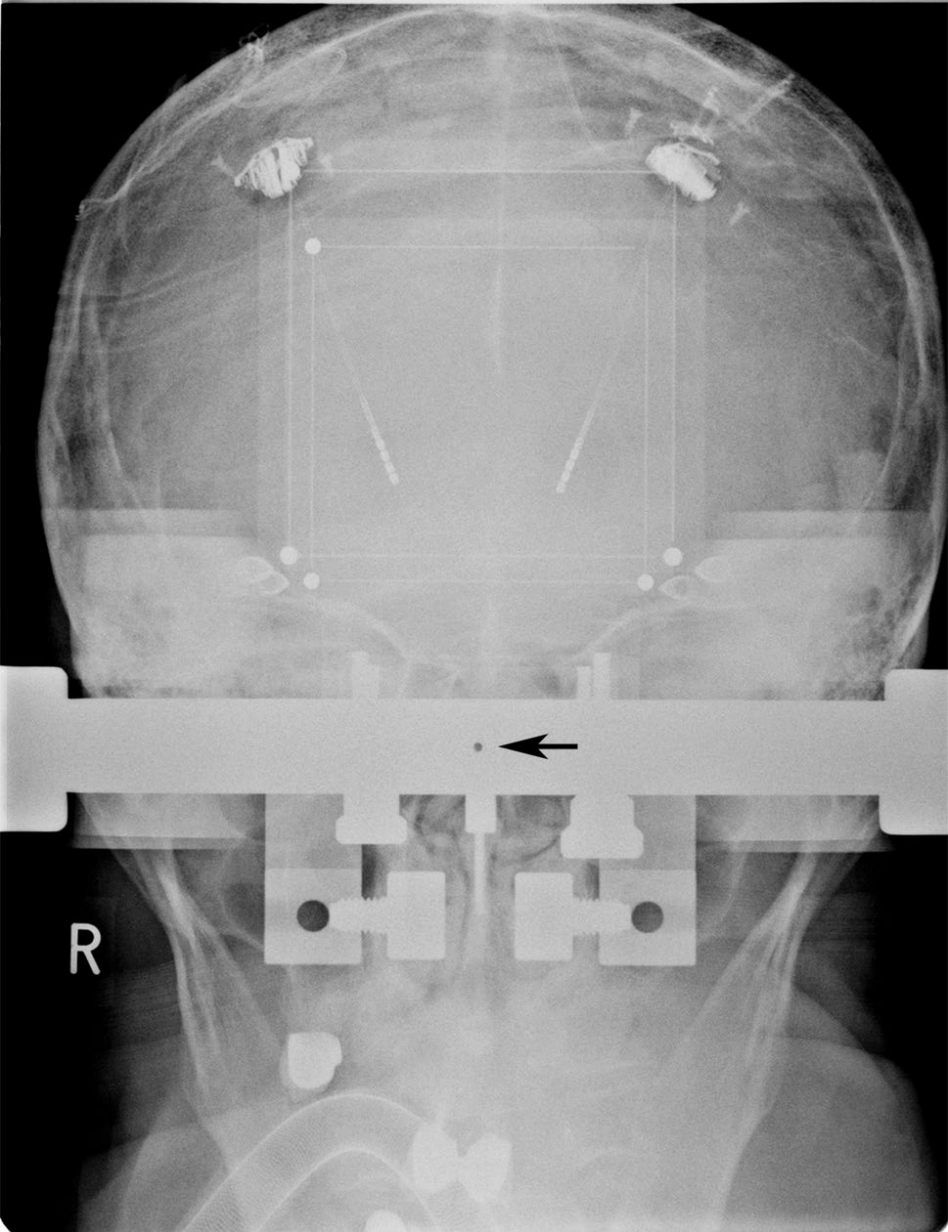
AP image obtained through a fixedly-mounted x-ray setting. The arrow points to the center of the image, where the X-ray beam is orthogonal
